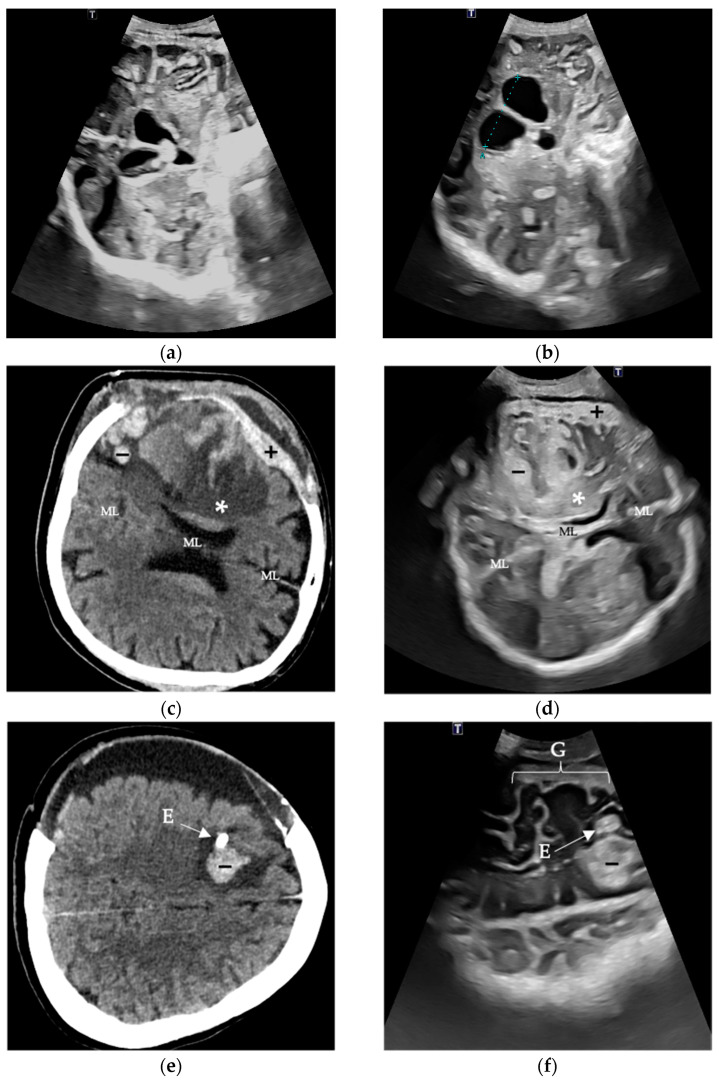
Evaluation of predefined image markers in selected cases In the first case, the coronal view of the lateral ventricles and the third ventricle with opened EVD (a) and progression in ventricular growth after 24 h EVD closure (b) is shown. In the case of a hemorrhagic infarction in a 65-year-old patient, both the corresponding cranial CT (c) and the follow-up TUS examination (d) reveal infarcted brain tissue (*), a subtle subdural hemorrhage (+), as well as intracerebral hemorrhagic components (−). A relevant midline (ML) shift is not present. Finally, a cranial CT (e) and TUS (f) of a 41-year-old patient with spontaneous SAH is depicted. In this case, in order of EVD (E) implantation, a consecutive tract hemorrhage occurred that was evaluated daily. Also, gyration (G) with corresponding external cerebrospinal fluid spaces could be assessed via TUS in the absence of postoperative hemorrhage.
